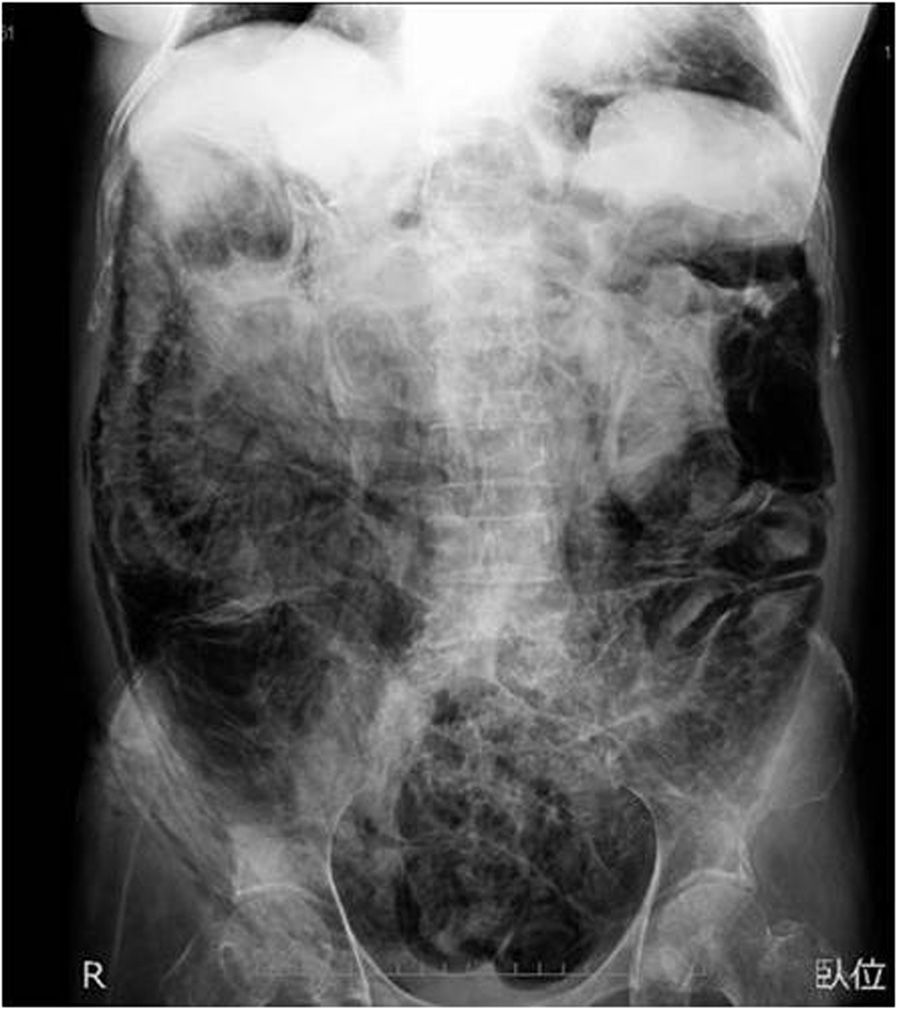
Abdominal X-ray performed before treatment revealed intestinal dilation and intraluminal gas
